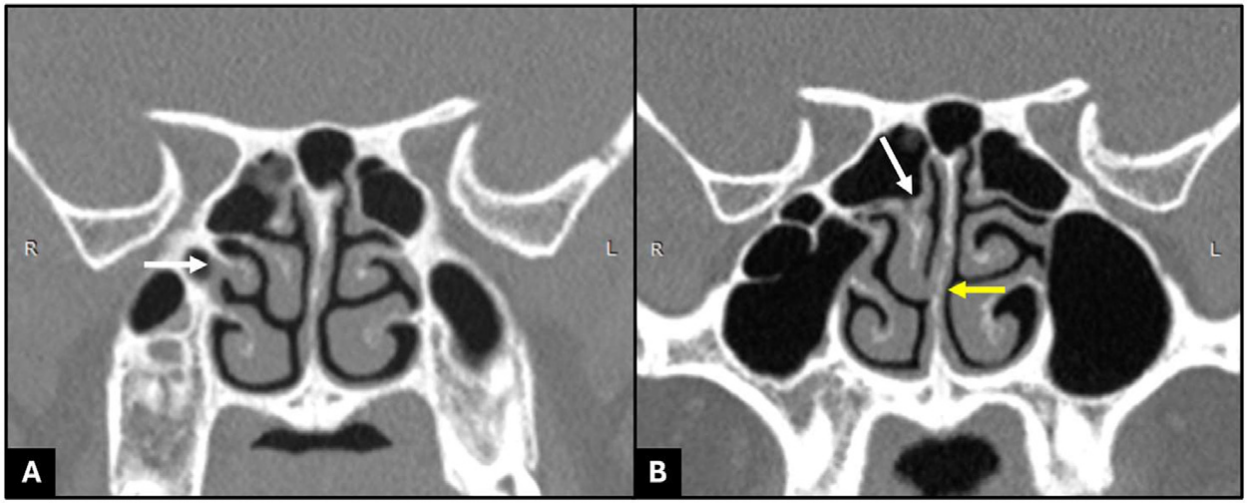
(A) A coronal CT scan of the paranasal sinus showed a right secondary middle turbinate (arrow). (B) The inferior side of the true (medial part) right middle turbinate attaches to the one-third inferior of the nasal septum (yellow arrow), and the superior side merges with the right superior turbinate (white arrow).
